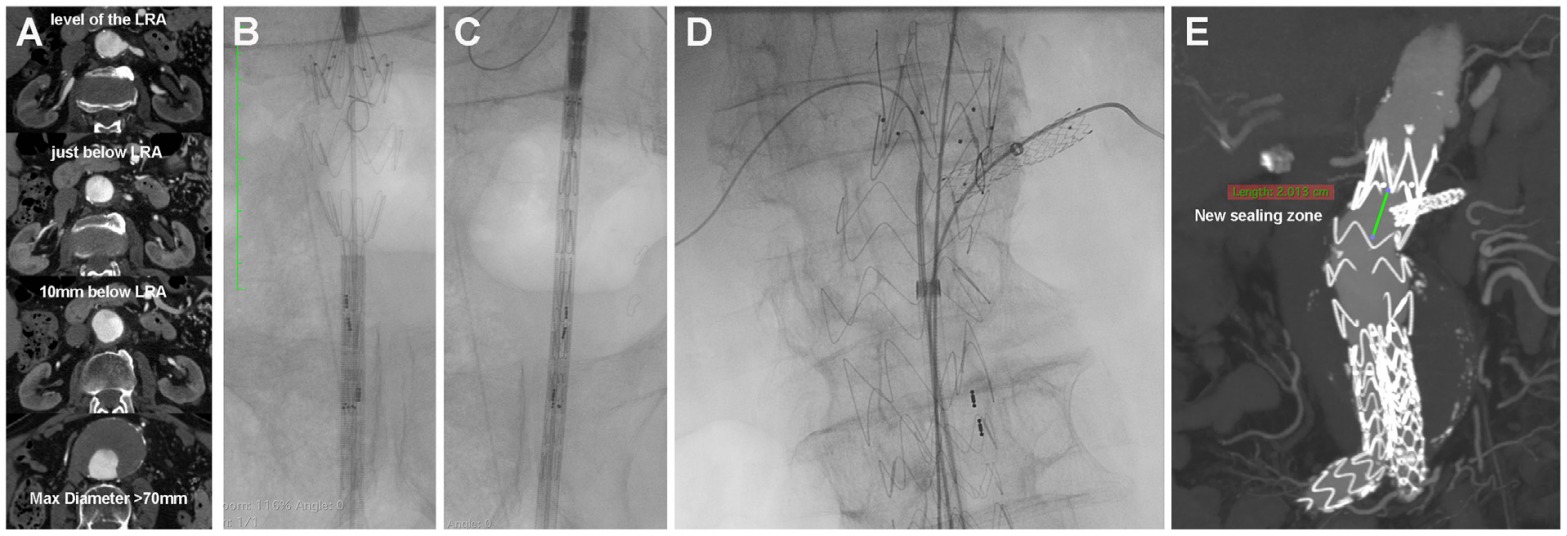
First single fenestration physician-modified TREO device implanted in our center showing the native preoperative computed tomography angiography images at the level of the left renal artery (*LRA*) and below, down to the maximum aortic diameter (A), confirming the lack of a proper infrarenal neck. The graft is partially unsheathed after modification under radiographic guidance (B) and then resheathed (C) and implanted (D) to provide a new sealing zone of 20 mm with implementation of a LRA fenestration (E).
